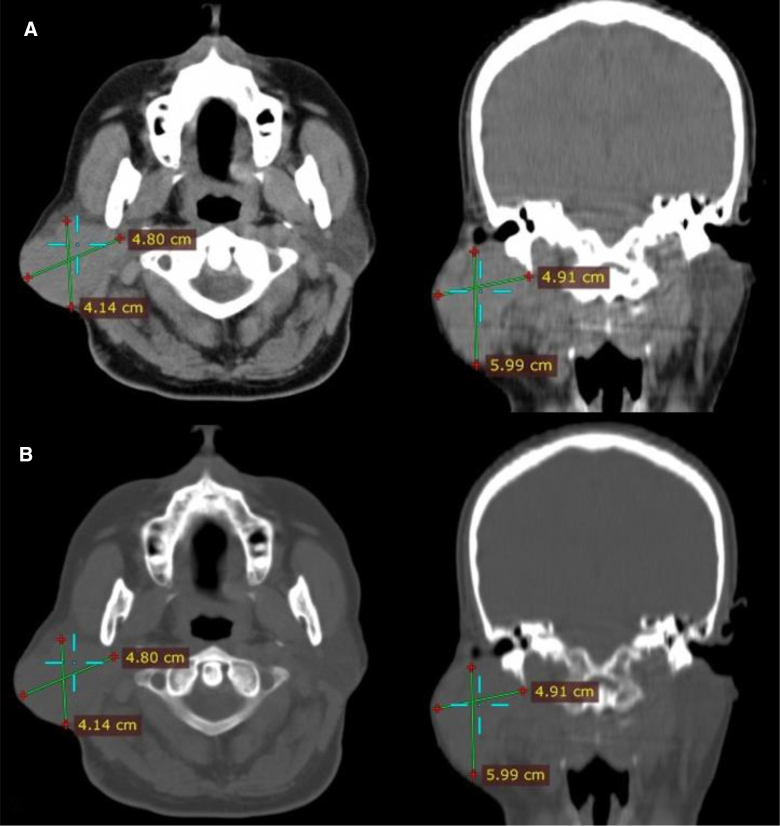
Axial and coronal CT scan revealing a homogeneous, moderately intense lesion in the superficial lobe of the right parotid gland. (A) Soft-tissue window. (B) Bone window. CT = computed tomography.
